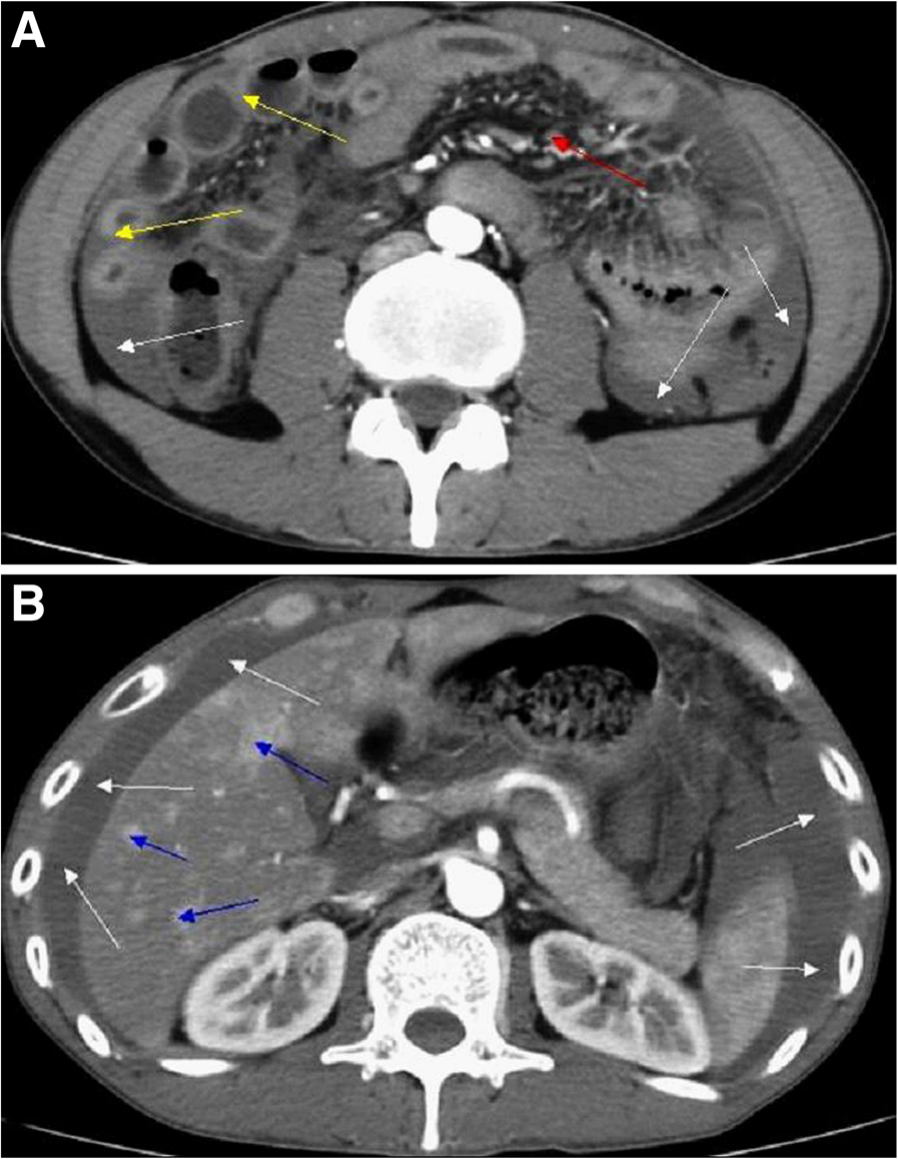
Enhanced computed tomography scan. (A) A significantly thickened intestinal wall (yellow arrows) located in the end of the jejunum and the proximal ileum, excessive ascites (white arrows) and a few enlarged lymph nodes in the abdomen (red arrow). (B) Different degrees of enhancement were noted in the hepatic arterial phase (blue arrows).
